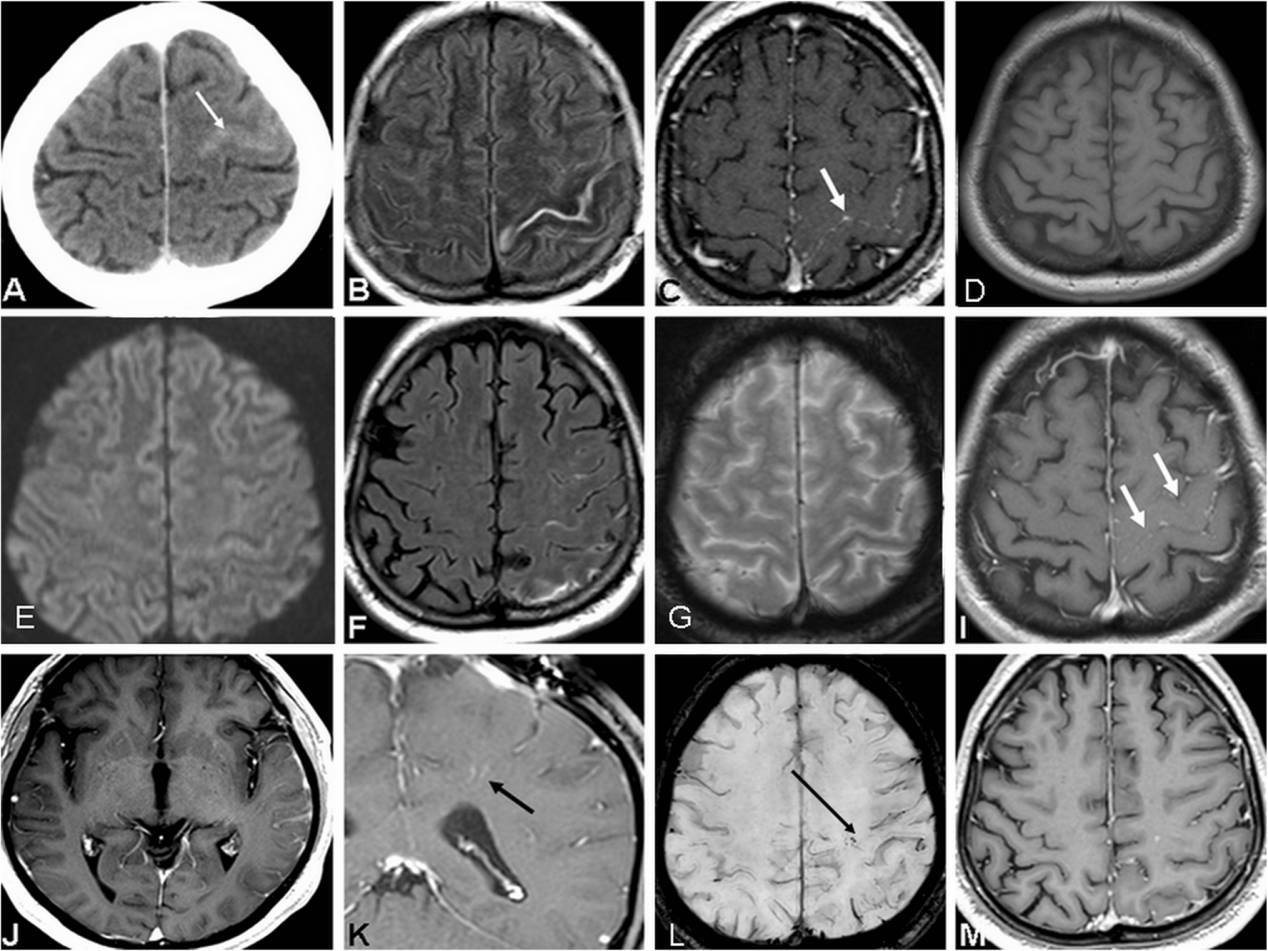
a–m Axial pre-contrast CT scan showed slightly hyperdense central sulcus (a, white arrow). Sulcal hyperintensity was detected on axial FLAIR image (b, white arrow). After contrast administration (c, white arrow), a leptomeningeal pattern of “dotted line” enhancement was observed, while on native T1W images (d) the sulcal narrowing was evident only. There was no restricted diffusion observed (e). Relapse occurred after 2 months, affecting postcentral, precentral sulcus (f), with no hemosiderin deposits on T2*W images (g) and the typical “dotted line” leptomeningeal enhancement (i, j, white arrows). Spread along perivascular spaces was observed as linear contrast enhancement along dilated veins (k, l, black arrows). After corticosteroid treatment, 6 weeks later, almost complete resolution was observed (m). A discrete dotted micronodular enhancement was still evident in precentral sulcus on the left, as well as along perivascular spaces in the left hemisphere
